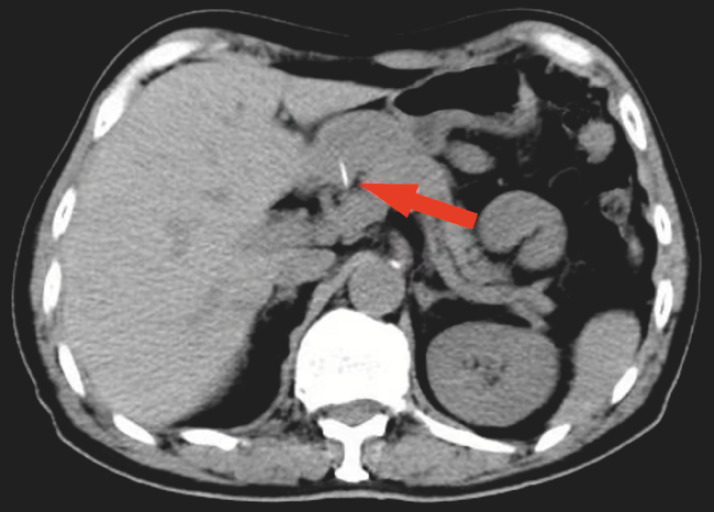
Abdominal computed tomography demonstrated a strip of high density shadow (arrow) in a patient presenting with epigastric discomfort of 1 month’s duration.
